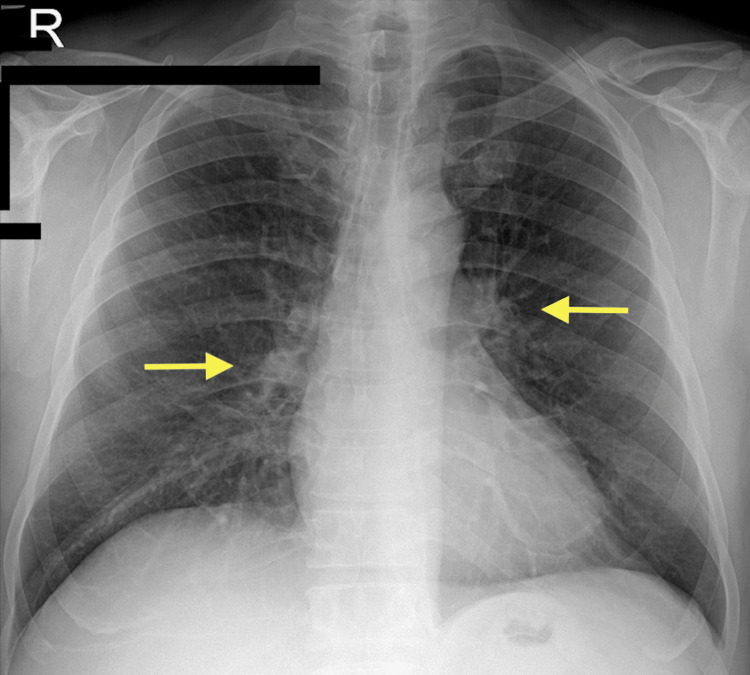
Hilar bronchiectasis (arrows) but no active infiltration on preoperative chest X-ray
